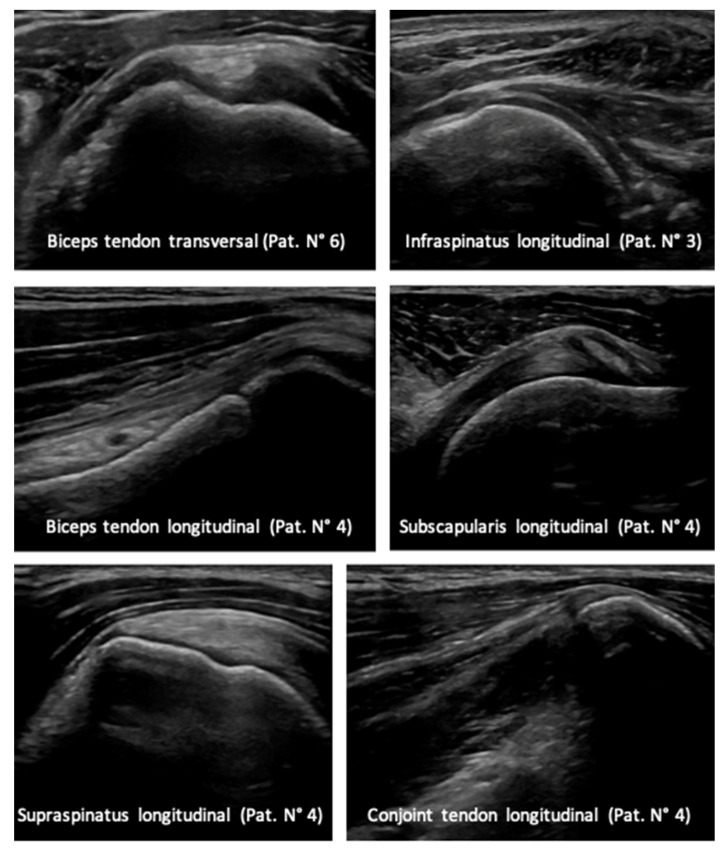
Sonographic scans 2 years postoperatively of the soft tissue surrounding the shoulder joint.
